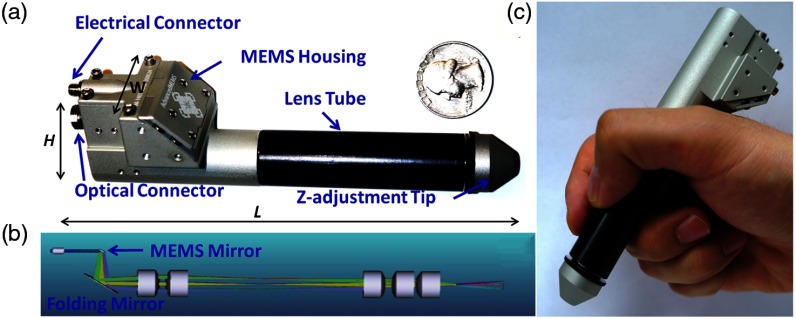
Intraoperative MEMS-based handheld Doppler OCT probe to observe microvascular anastomosis. (a) CAD design of handheld probe compared to US currency (quarter). (b) Interior optical design within probe. (c) Handheld probe in hand. Reprinted with permission.^168^
